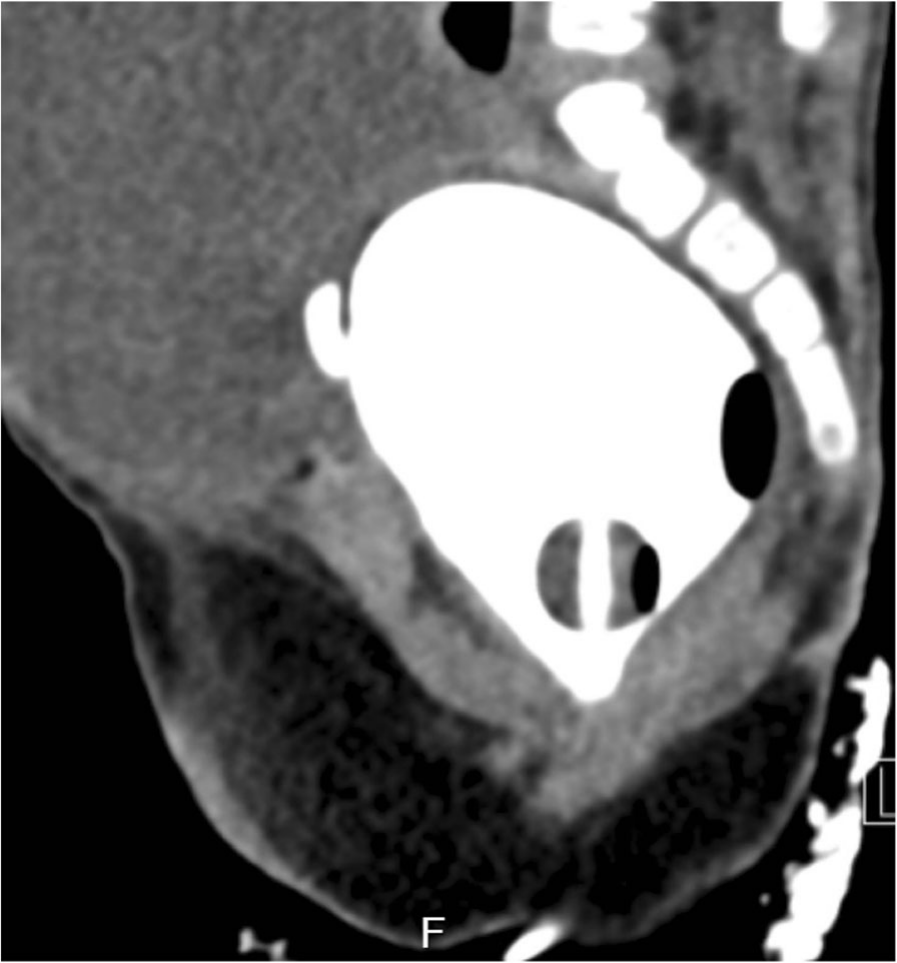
CT cystogram, showing no leak of contrast. The contrast opacified structure had similar appearance to that seen on the MCUG, however, its location was thought to be lower and more posterior than the normal location of a full urinary bladder. Thus this structure was thought to possibly represent the vagina instead
